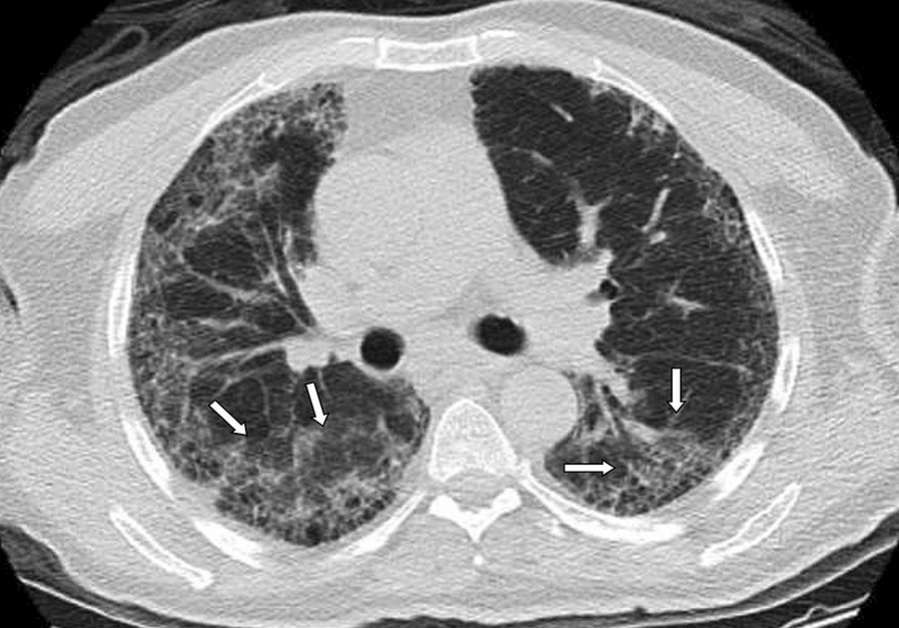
Case example defined in the indeterminate for the BSTI guidance statement, and in the typical category for the RSNA expert consensus statement. An axial chest CT image of a 68-year-old male without COVID-19 shows peripheral multifocal GGOs (arrows) in the background of moderate emphysema. The final diagnosis was usual interstitial pneumonia
